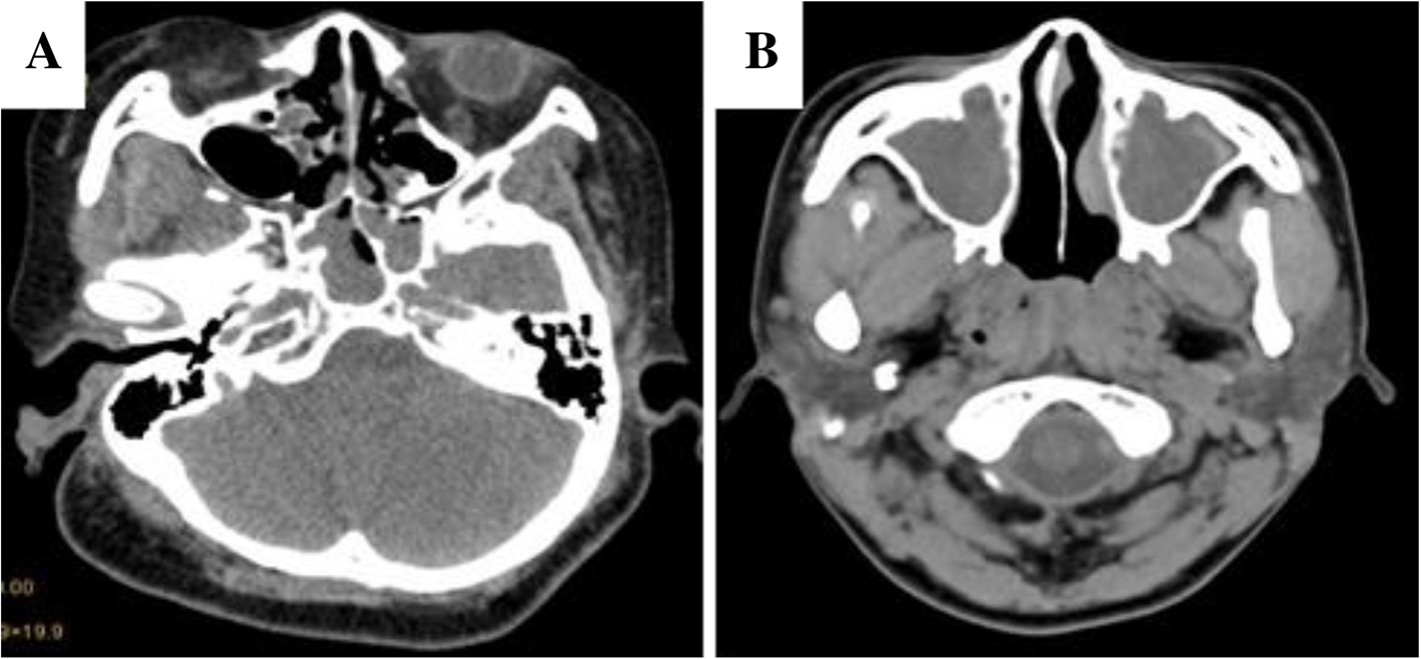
Computed tomography scans of REAH. A. Axial CT showing soft tissue masses in both nasal cavities, mucosa in maxillary sinus, ethmoid sinus thickening in some extent. B. Additional CT of maxilla. Additional CT Note occlusion of right posterior choana and nasopharynx by mass.
